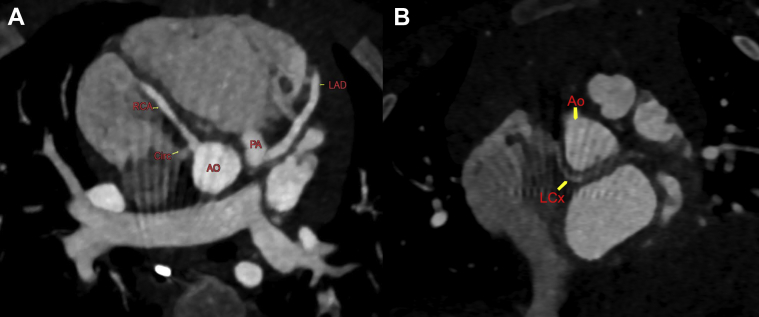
Computed Tomography Detailing Anomalous Coronary Anatomy (A) Reformatted oblique axial computed tomography showing all coronary arteries with clear anomalous left anterior descending coronary artery from pulmonary artery anatomy. (B) Left circumflex (Circ or LCx) artery is seen originating from the proximal RCA with course posterior to the aorta. Abbreviations as in Figure 1.
